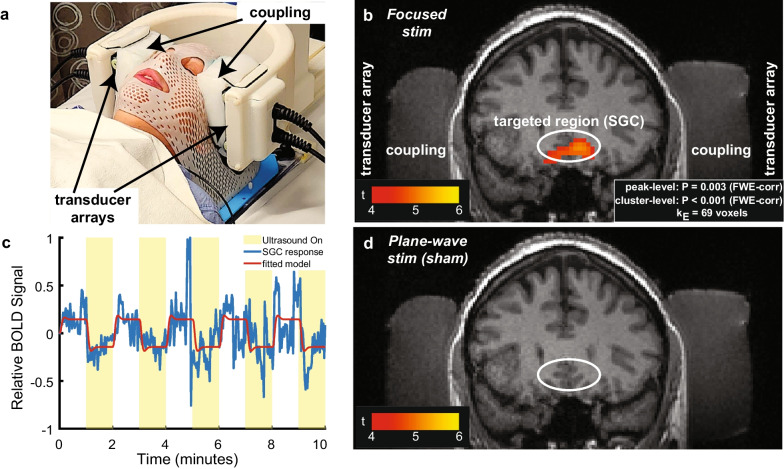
Approach for effective deep brain ultrasonic neuromodulation in humans. a Application to a patient with major depression. Programmatic electronic focusing is achieved using two sets of 126 individually controlled transducer elements, one over the left and one over the right side of the head. The subject’s head is secured in place using a standard radiological mask. Lateral windows are made within the mask for unobstructed ultrasound propagation. Coupling is mediated using cryogel. b Treatment validation. A standard Siemens flex coil was positioned over the subject’s head. Ultrasound was delivered into the target in 30-millisecond on periods (650 kHz, 1.0 MPa peak pressure) followed by 4-second off periods (0.8% duty). The on and off periods were presented in 1 minute ON blocks, followed by 1 minute OFF blocks of no ultrasound, for a total of up to 10 min (see also c). The MRI scanner acquired fMRI BOLD signals during the stimulation. The colorbar shows the t-statistic associated with the BOLD difference between the ON and OFF blocks. The white circles outline the approximate location of the SCC. c The modulation of the blood-oxygen-level-dependent (BOLD) signal by the ON and OFF ultrasound conditions. The fitted model (red) assumes standard hemodynamic response. d Control stimulation. To control for potential generic artifacts associated with ultrasound, we delivered a stimulus that had the same waveform and pressure amplitude as the stimulus focused into the SCC, but was unfocused (that is, the transducers emitted a plane wave)
